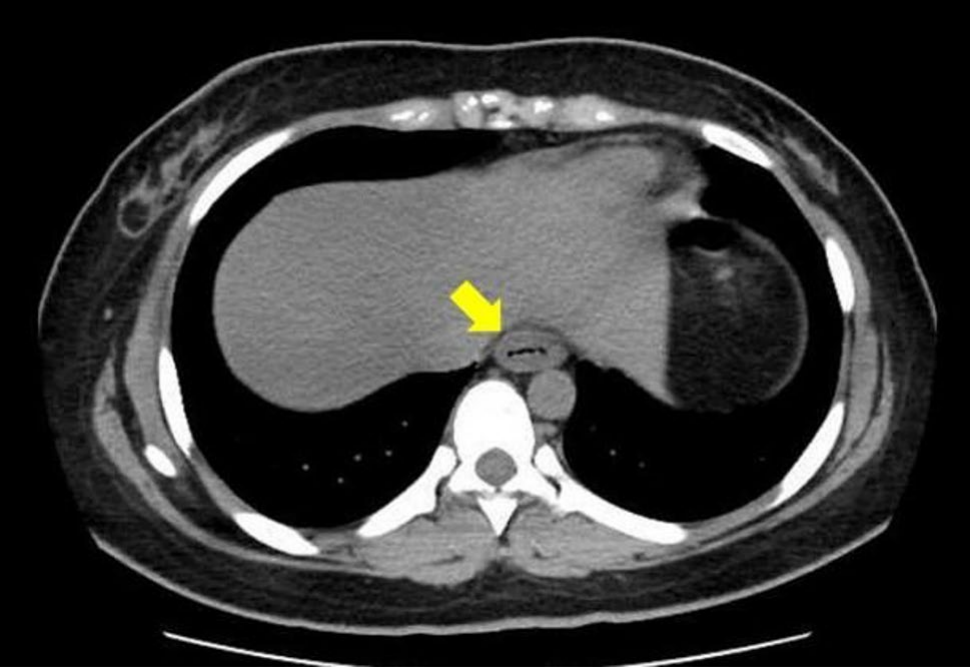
An abdominal axial computed tomography scan revealed concentric esophageal wall thickening (indicated by the arrow)
